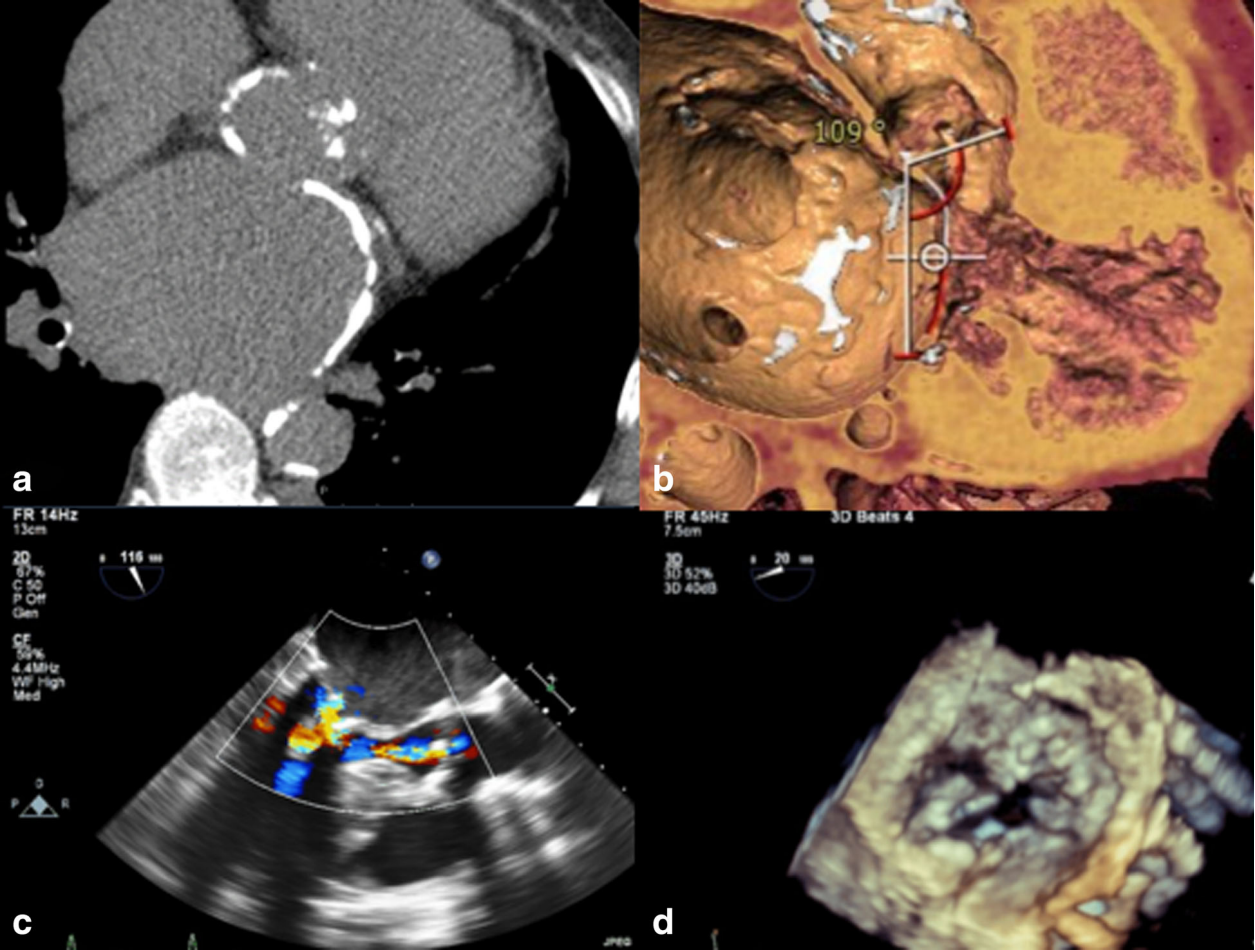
a Pre-operative CT-Scan: Severe calcification and dilation of *left atrium*. b CT-reconstruction with volume-rendering of the *left-ventricular* outflow tract: aorto-mitral angle of 109°. c TEE LVOT view in diastole, showing severe mitral valve stenosis and narrowing of the LVOT due to bulging of the inter-ventricular septum. d TEE 3D reconstruction of the native mitral valve during diastole showing severely decreased residual effective orifice area
